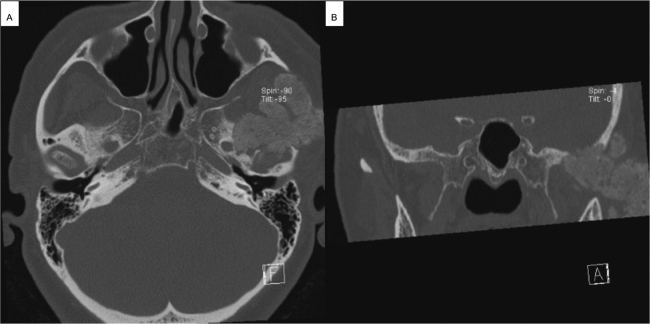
(A) Axial CT scan showing the osseous tumor of the left parotid gland reaching the foramen ovale of the skull base. (B) Coronal CT scan showing the tumor of the left parotid gland with osteodestruction of the left middle cranial fossa.
